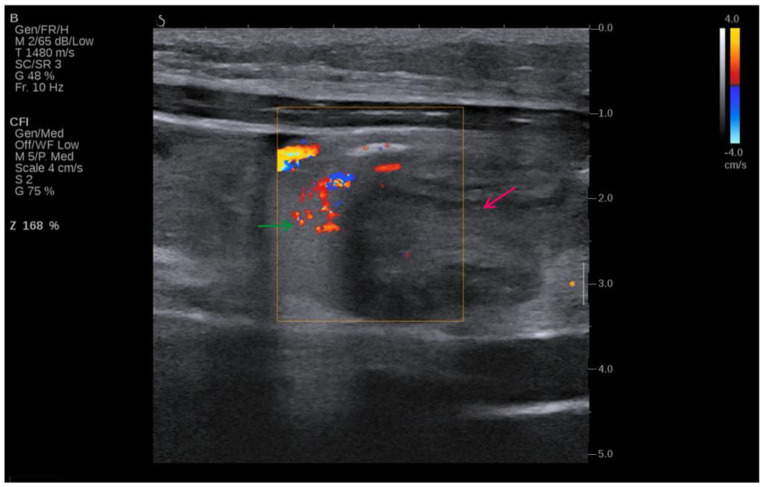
Ultrasound image of the volvulus—intestine twists around itself and its mesentery (red arrow), “whirlpool sign” (green arrow).
